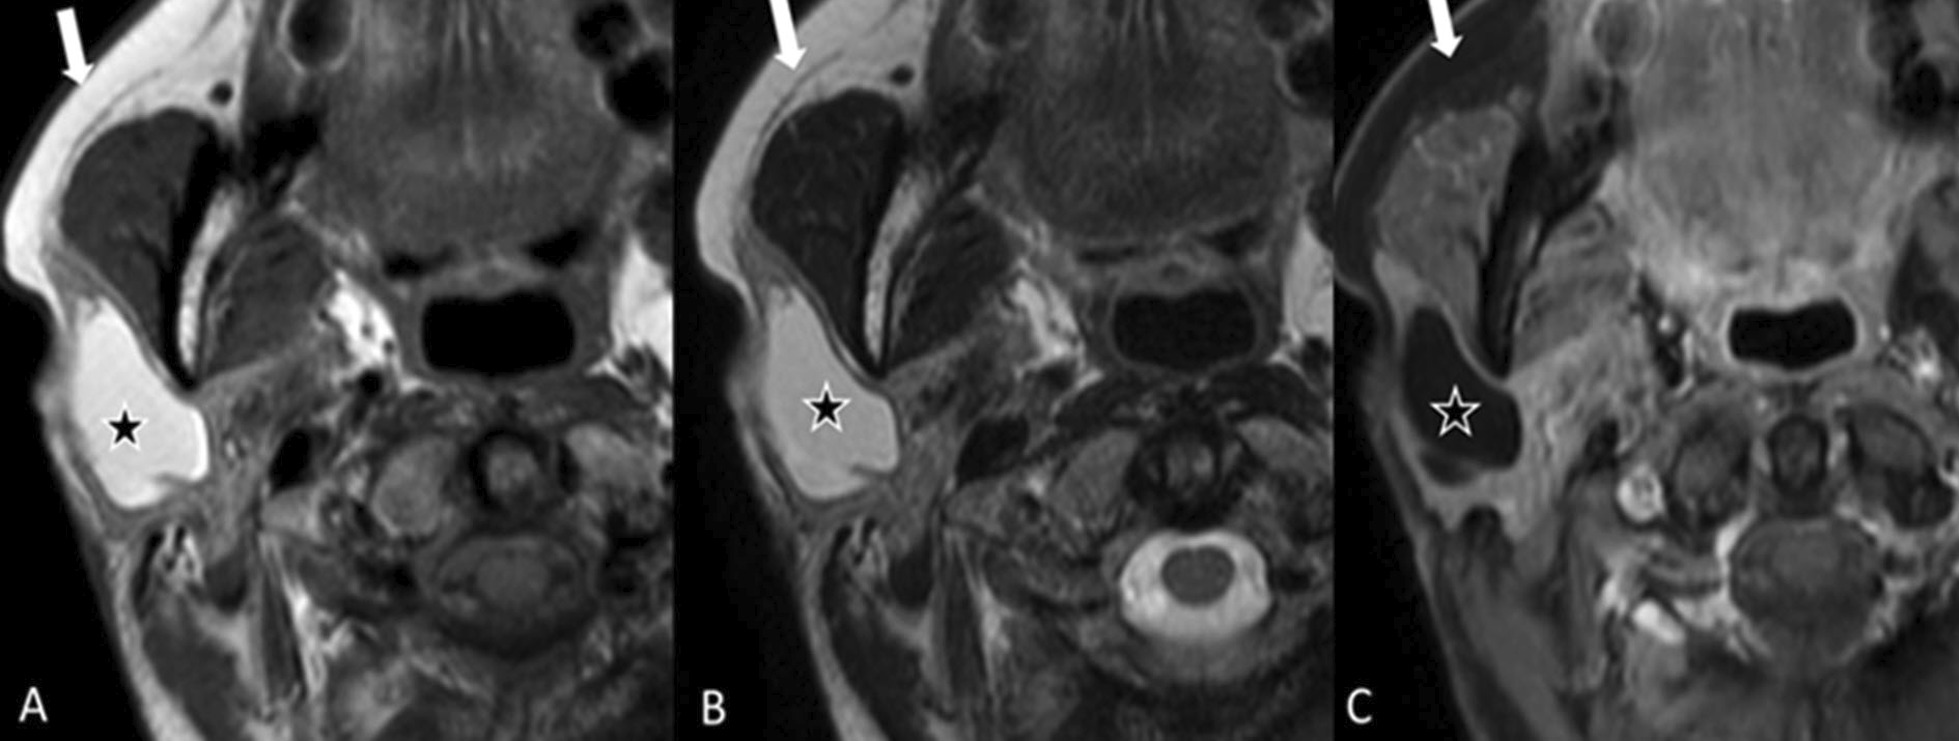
Lipoma. An 82-year-old female with an incidental finding of lipoma. Axial T1‑w (A) and axial T2‑w (B) MRI demonstrates a well‑circumscribed high signal lesion in the superficial lobe of the right parotid gland (white arrows) similar to that of subcutaneous fat. Axial T1‑weighted post-contrast fat‑suppressed MRI (C) shows lack of enhancement with a typical hypointense signal isointense to fat (white arrow)
